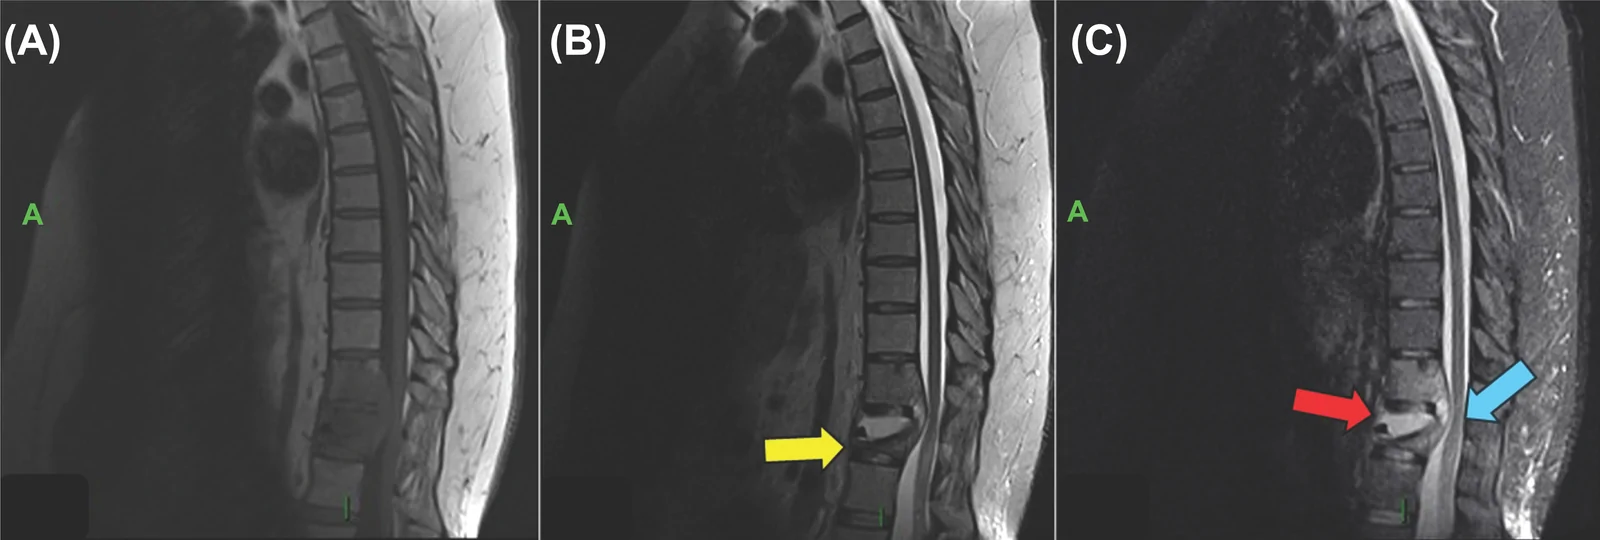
Thoracolumbar MRI demonstrating sagittal, T1-weighted (A), T2-weighted (B), STIR (C). Marked T12 height loss with anterior wedging (yellow arrow) and cement from prior vertebroplasty (red arrow). Epidural phlegmon/abscess produces ≈60% canal narrowing with features of compressive myelopathy (blue arrow). Abbreviations: MRI, magnetic resonance imaging; STIR, short-tau inversion recovery.
